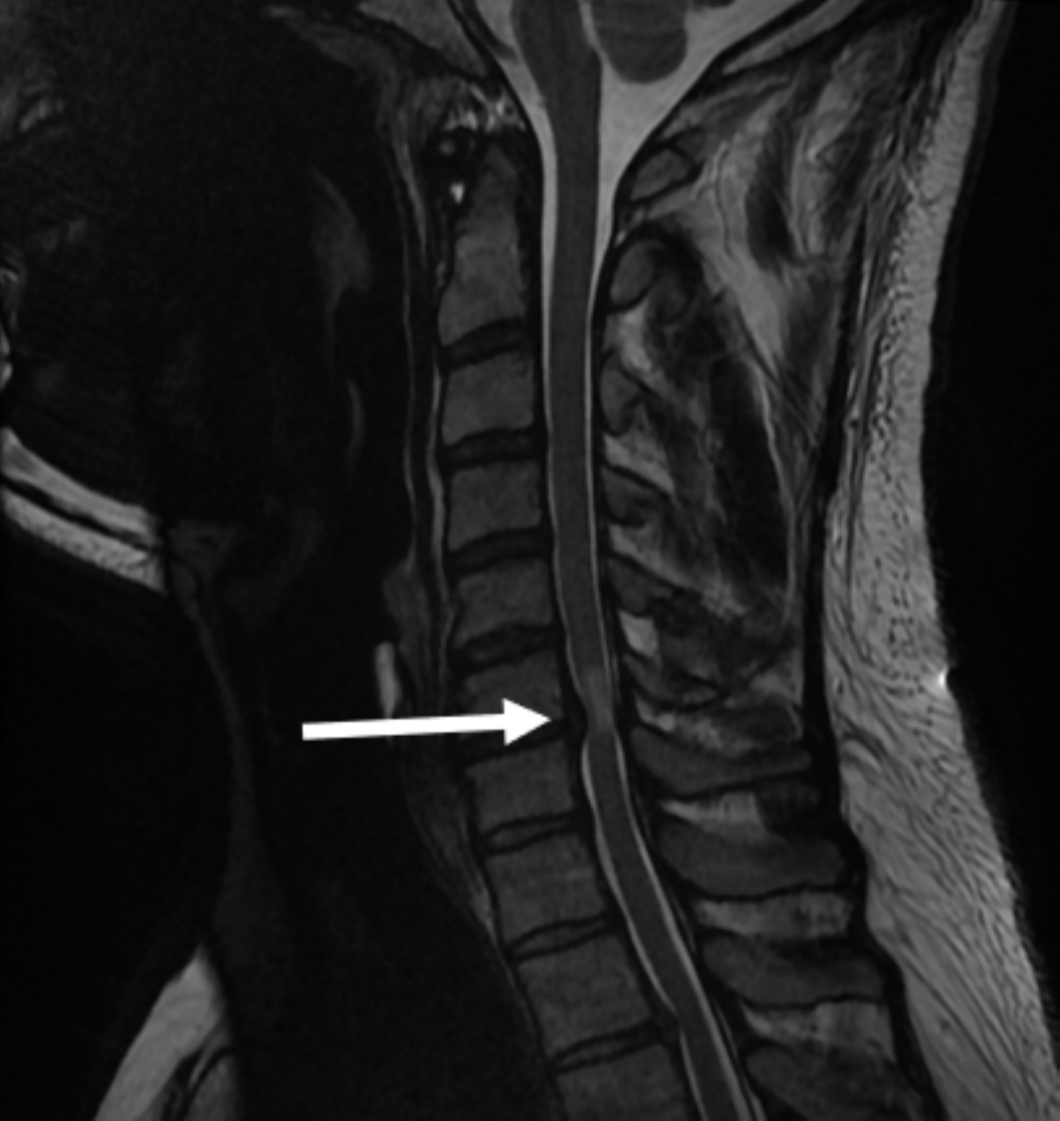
T2-weighted MRI showing spinal cord compression and focal cord oedema at the C6/7 level
